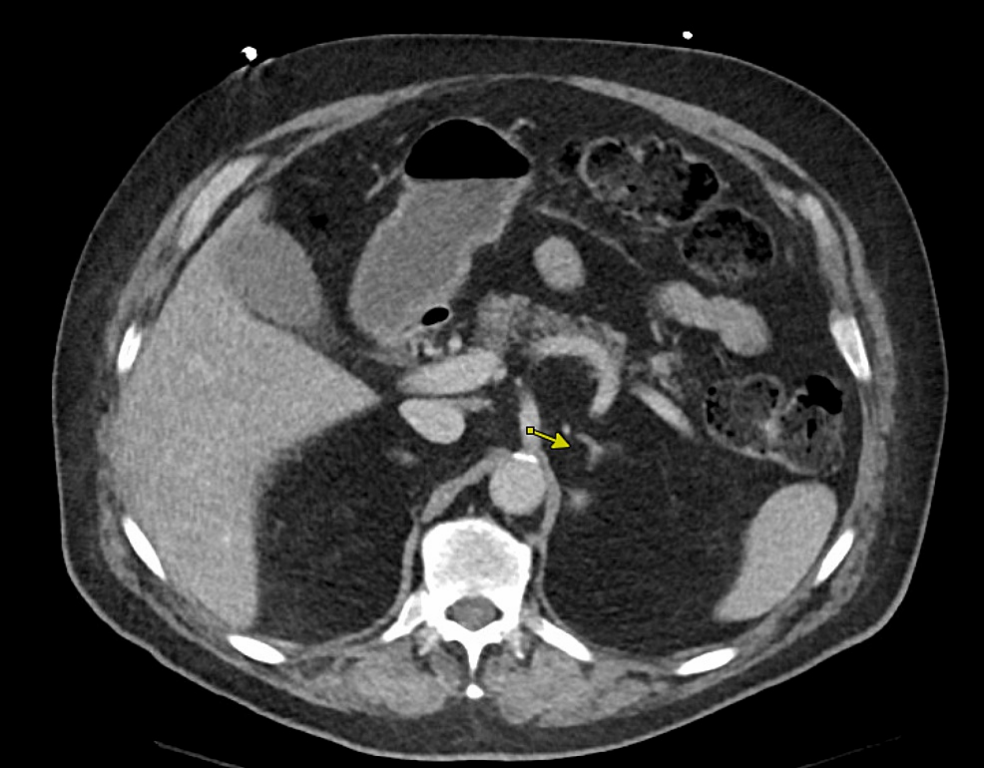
CT imaging of the left adrenal gland (arrow). CT: computed tomography
